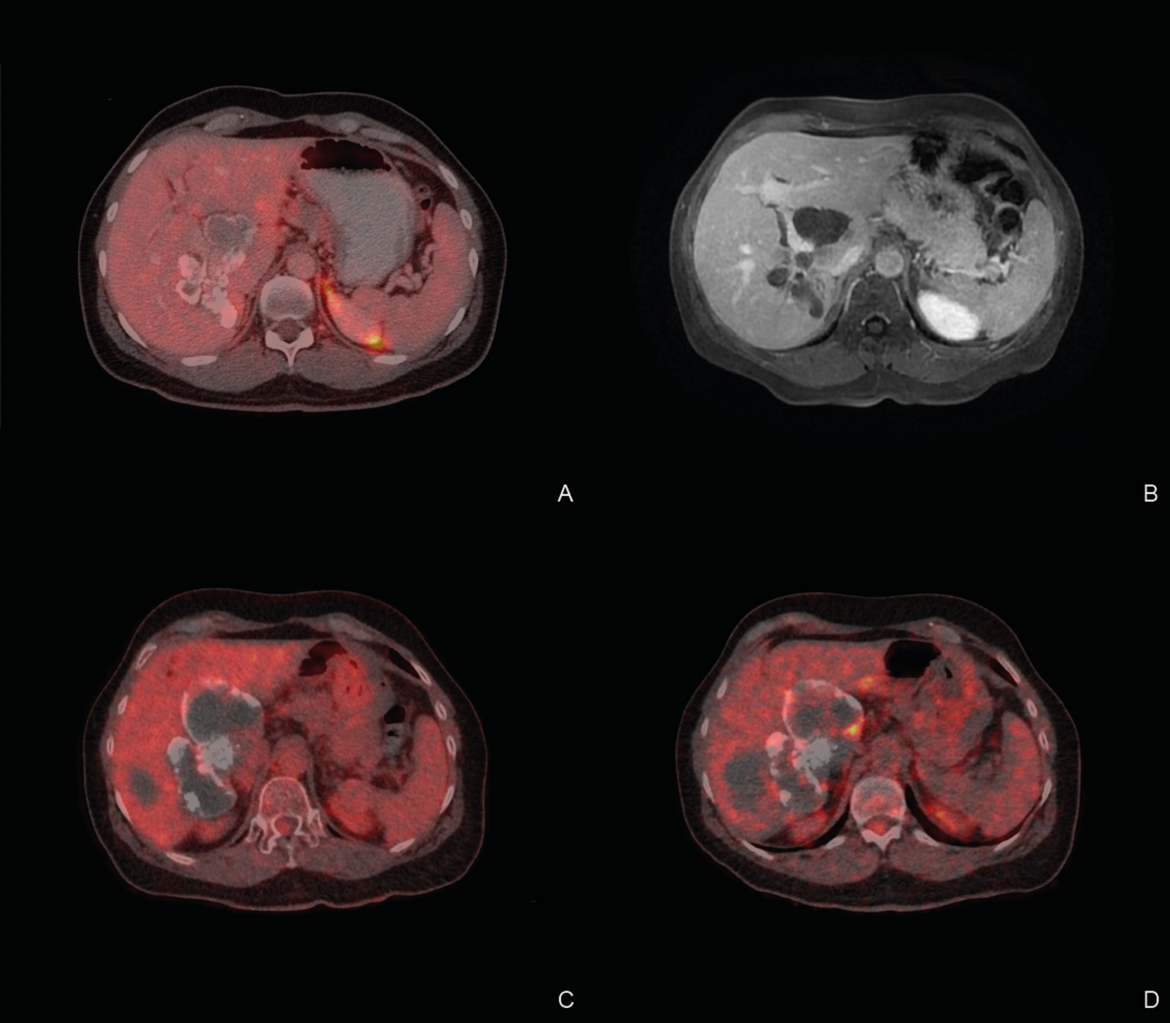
Imaging follow-up of the only patient that demonstrated a progressive disease course after cessation of benzimidazole treatment. A: PET-CT at baseline. B: MRI after 4 years off therapy. C: PET-CT after 14 years off-therapy. Imaging 1h after FDG injection. D: Same PET-CT as in C but imaging 3h after FDG injection. Note the discret hilar FDG uptake.
